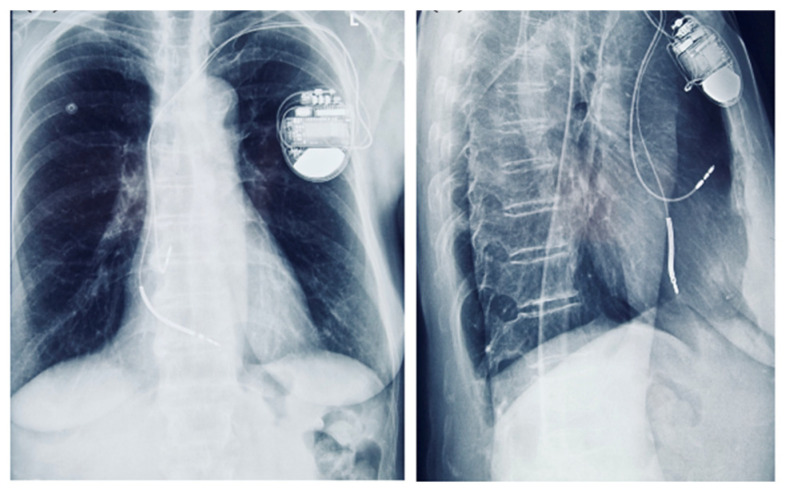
Chest radiograph showing a dual-chamber implantable cardioverter-defibrillator (ICD) in anteroposterior and lateral views.
